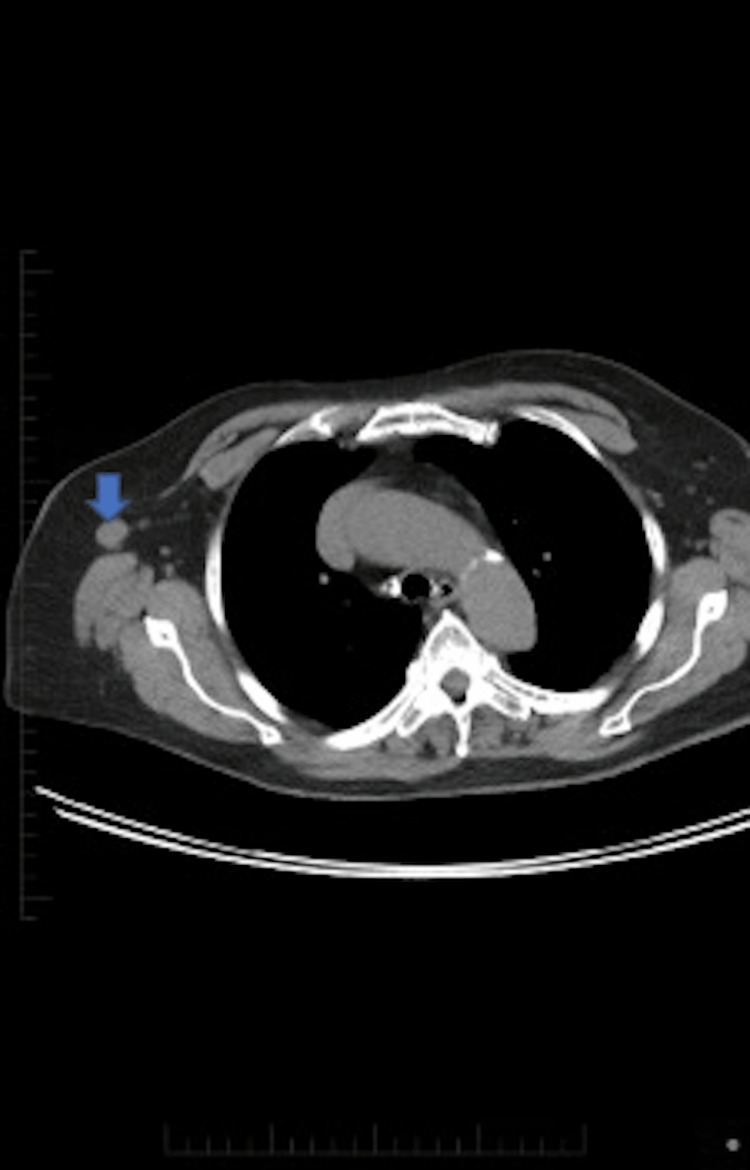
PET-CT with approximately 1.5 cm axillary lymph node. PET-CT: positron emission tomography-computed tomography
